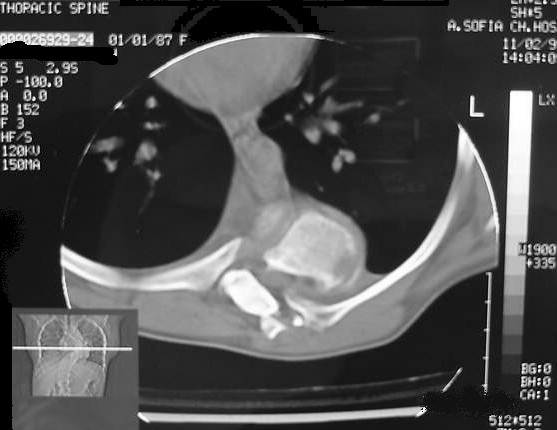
Preoperative CT scan at the level of T8 shows intraspinal dislocation of the left eighth rib head through neural foramen.
